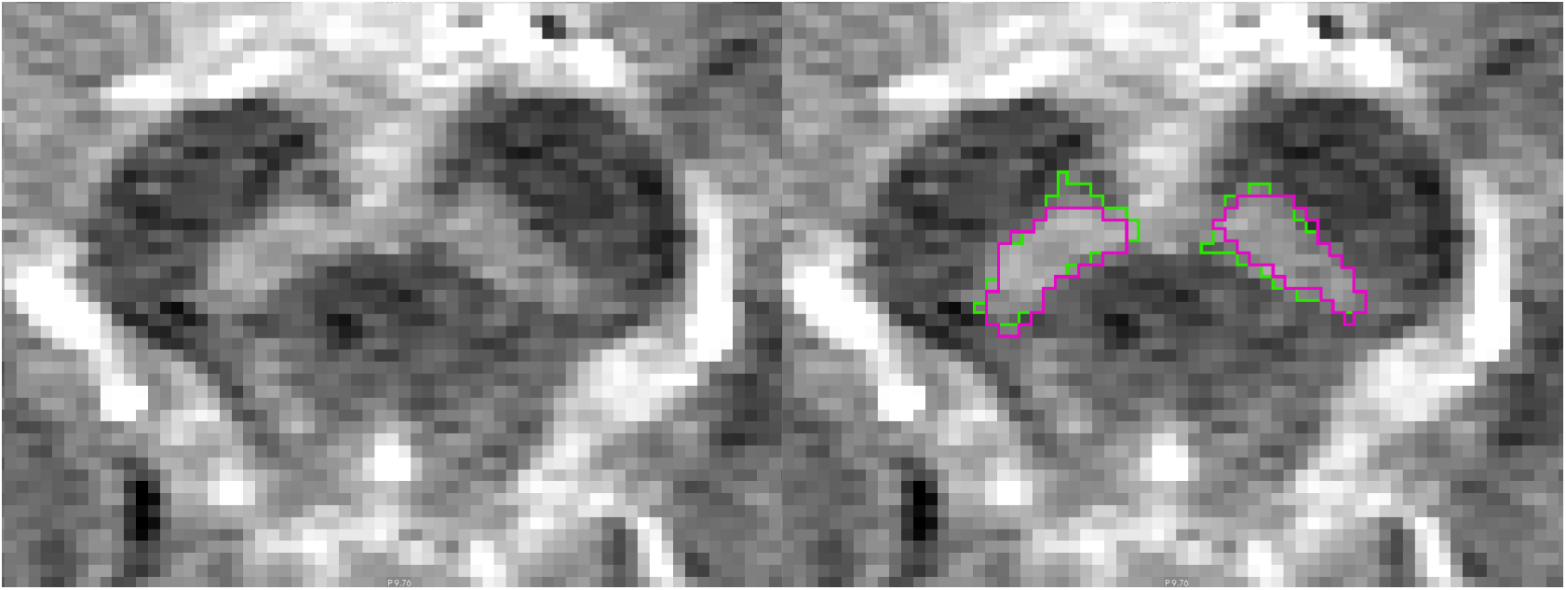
NM-MRI of the midbrain (axial slice) with outlined masks of the substantia nigra hyperintensity from the two raters on the right.
